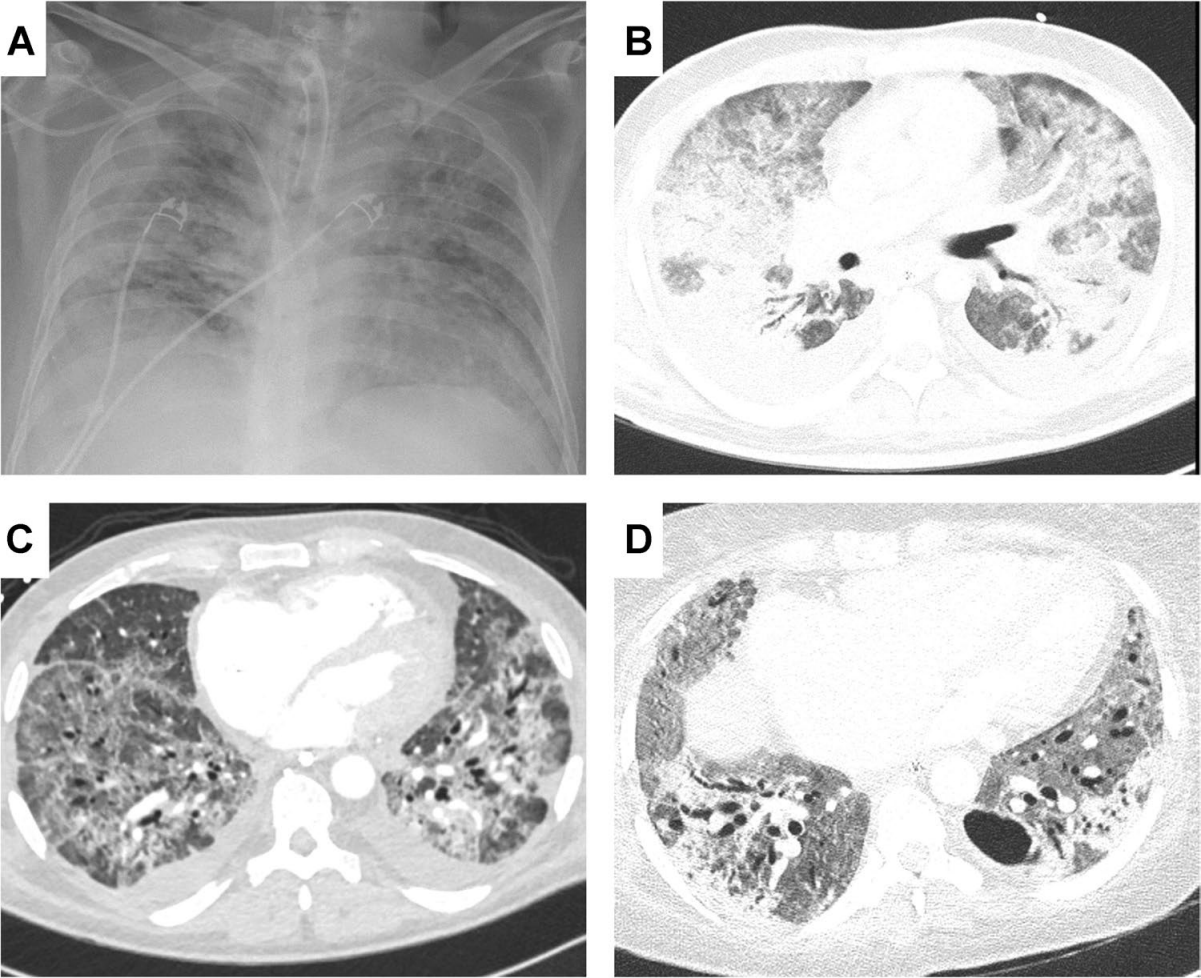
Radiographic images of pulmonary changes in COVID-19 over time: A Typical radiographic presentation of acute respiratory distress syndrome with bipulmonary infiltrates, so called “white lung”. B CT-image of a 39-year-old previously healthy man after 30 days of extracorporeal membrane oxygenation (ECMO) treatment displaying bipulmonary ground glass opacities and basal infiltrates in line with (prolonged) ARDS. C CT-image of a 62-year-old man showing interstitial fibrosis and cystic remodeling including subpleural areas after 3 weeks of ECMO treatment. D CT-image of 34-year-old woman after 70 days of ECMO treatment with advanced pulmonary remodeling
